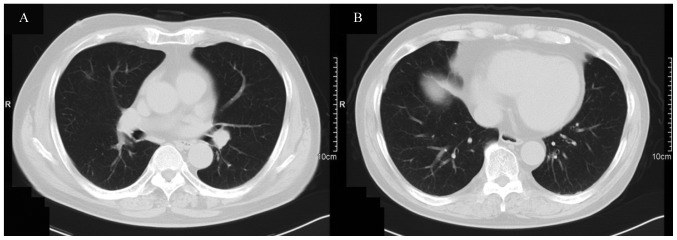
Chest computed tomography following nine courses of therapy. There is no metastatic tumor in the (A) right lung or (B) left lung.
